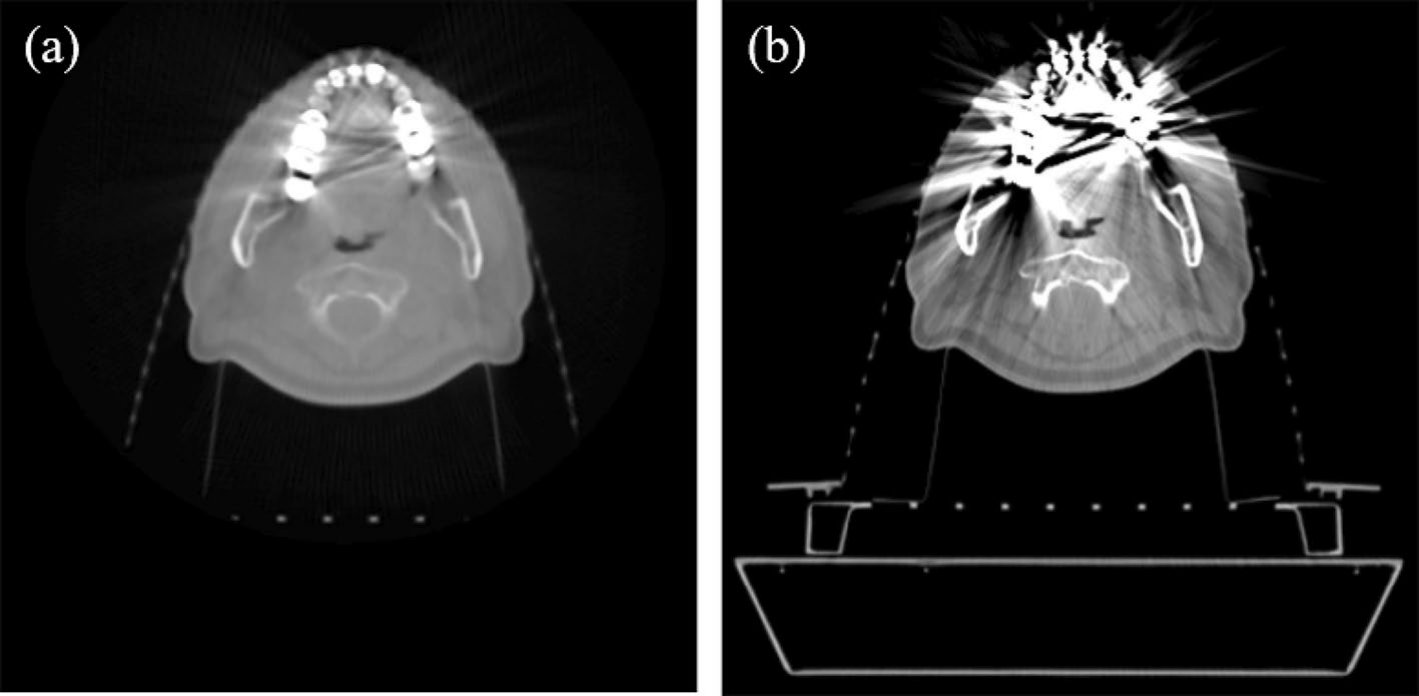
MV-CBCT images (a) and kV-CT images (b) of same slice of same patient.
